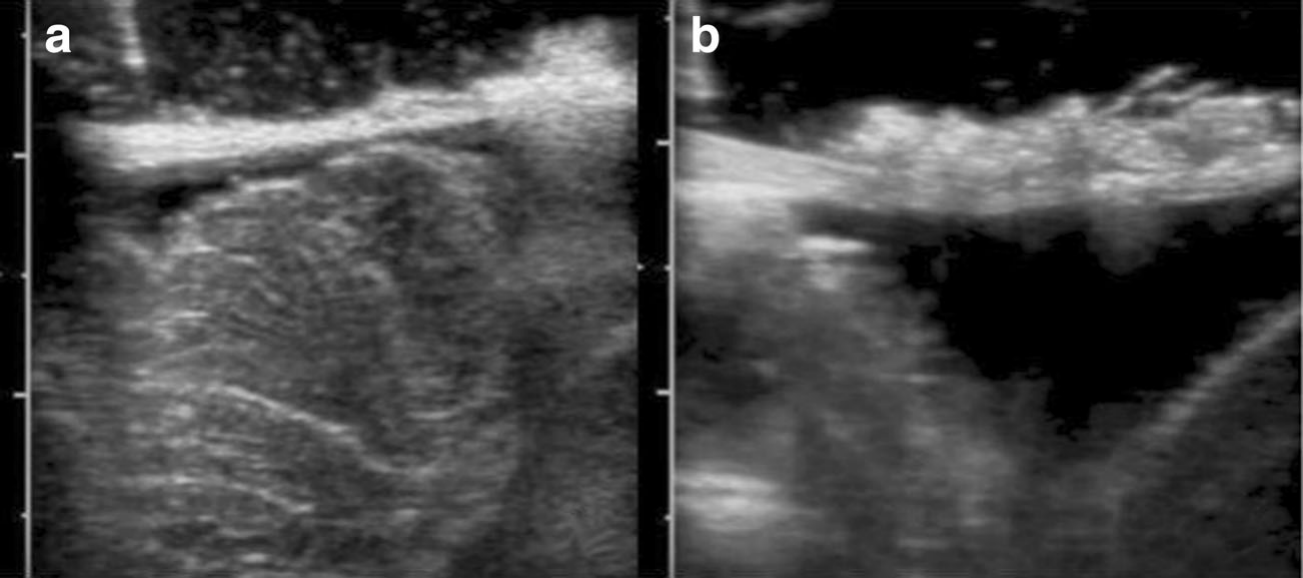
a Intraoperative ultrasound demonstrating the cerebellar tonsil prior to durotomy and subpial resection. b Intraoperative ultrasound after MIST is performed to confirm adequate tonsillar resection
